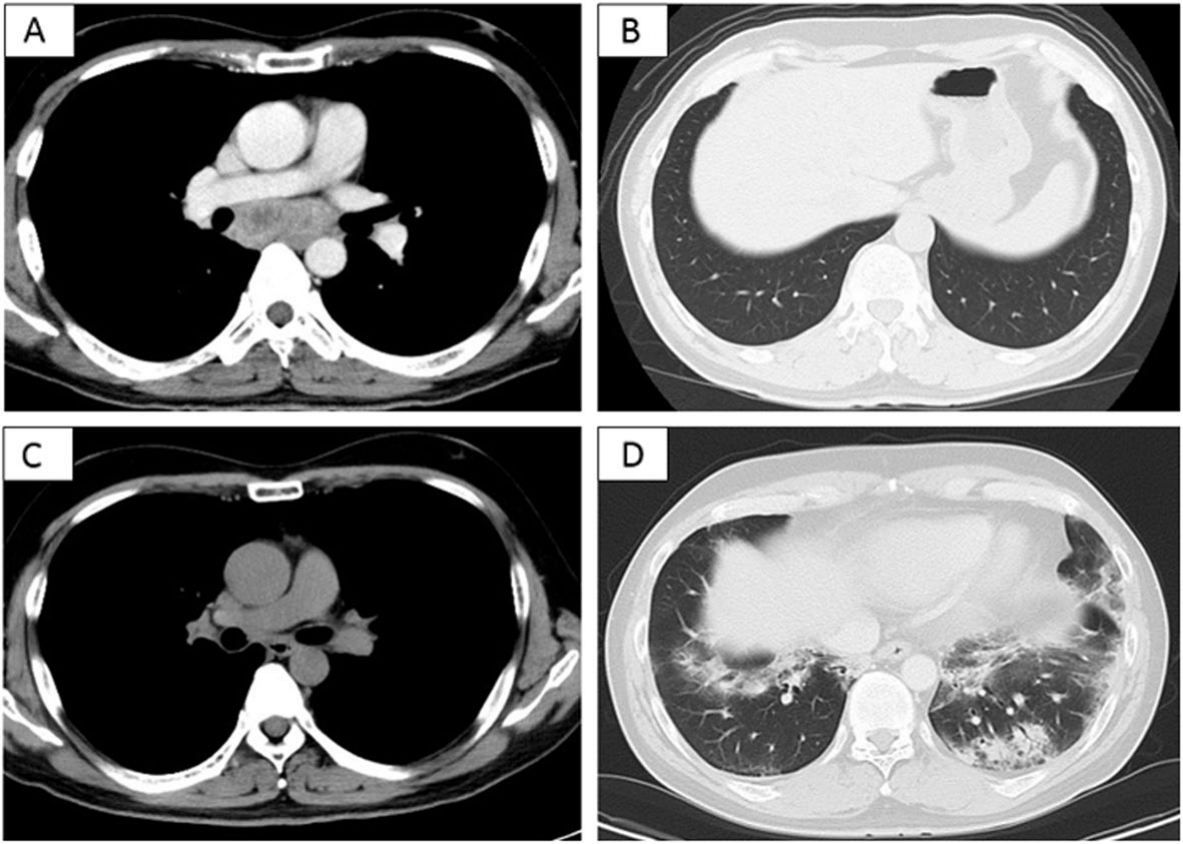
Progress of chest computed tomography (CT) images: A-B Chest CT findings before atezolizumab administration. C-D Chest CT findings on admission. Reduction of mediastinal lymph nodes was observed, but peribronchial consolidation appeared
